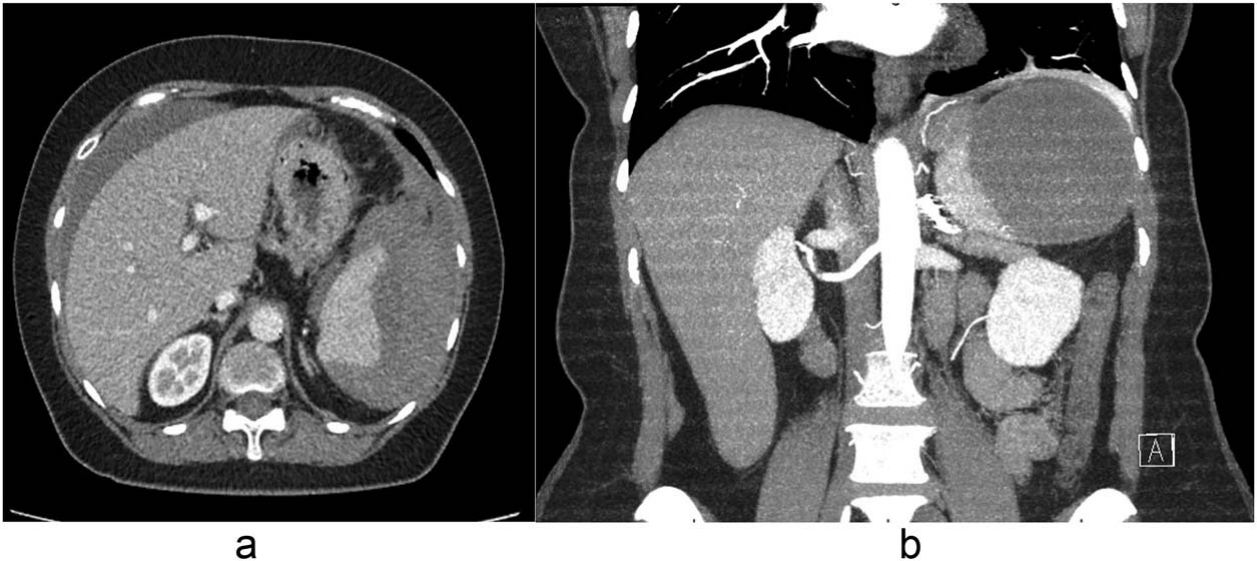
(a) Axial and (b) coronal contrast-enhanced CT images demonstrating a peri-splenic hematoma measuring 8.6 × 10.2 cm with associated hemoperitoneum.
